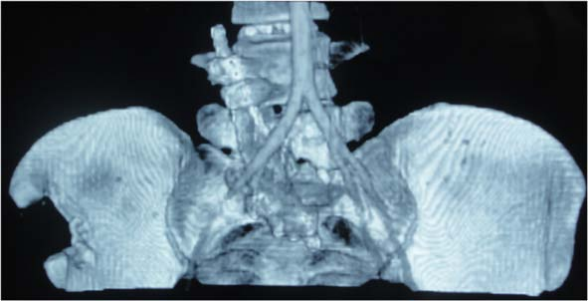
Postoperative computed tomographic angiography (CTA) showing iliac artery adjacent to the lumbosacral spine at the 24 months’ follow up
